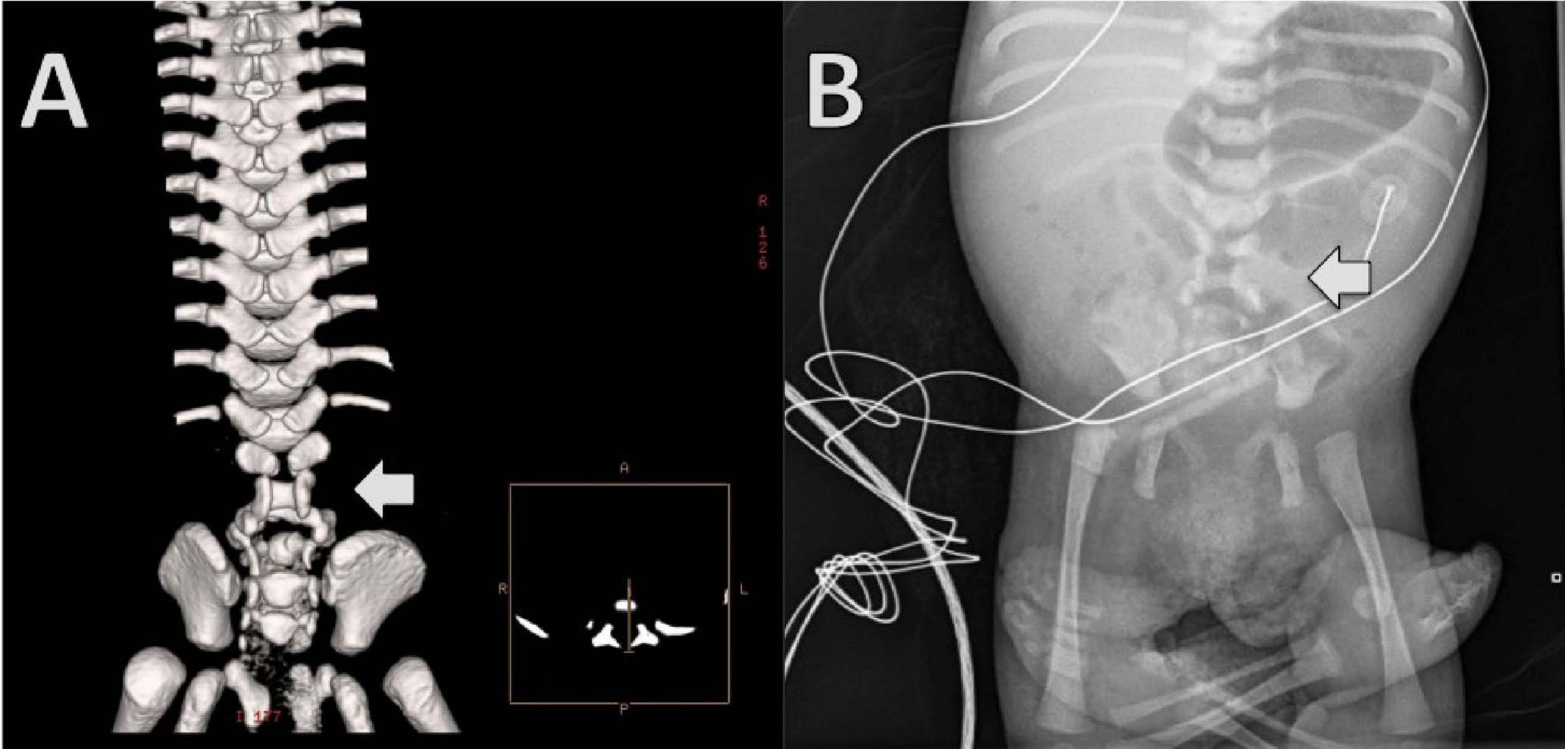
(A) Computed tomography 3-dimensional spine reconstruction and (B) chest and abdominal x-ray show multilevel segmental spinal dysraphism involving the lumbar and sacral levels with hypoplastic vertebral elements. The most affected region of lumbar spine is indicated by arrows.
